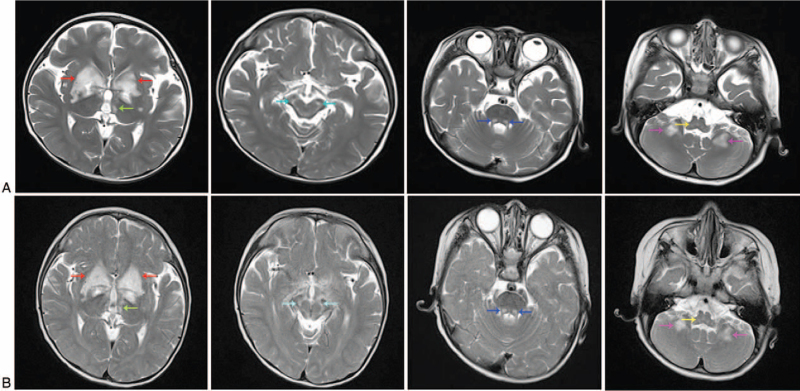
A. Head MRI showing the lesions of the child before treatment. B. Head MRI showing lesions more than 1 month after mitochondrial cocktail treatment. Red arrow: bilateral basal ganglia; green arrow: thalamus; blue arrow: cerebral peduncle; purple arrow: pons; yellow arrow: medulla oblongata; magenta arrow: dentate nucleus. There was no significant change in the head MRI findings after mitochondrial cocktail treatment. MRI = magnetic resonance imaging.
